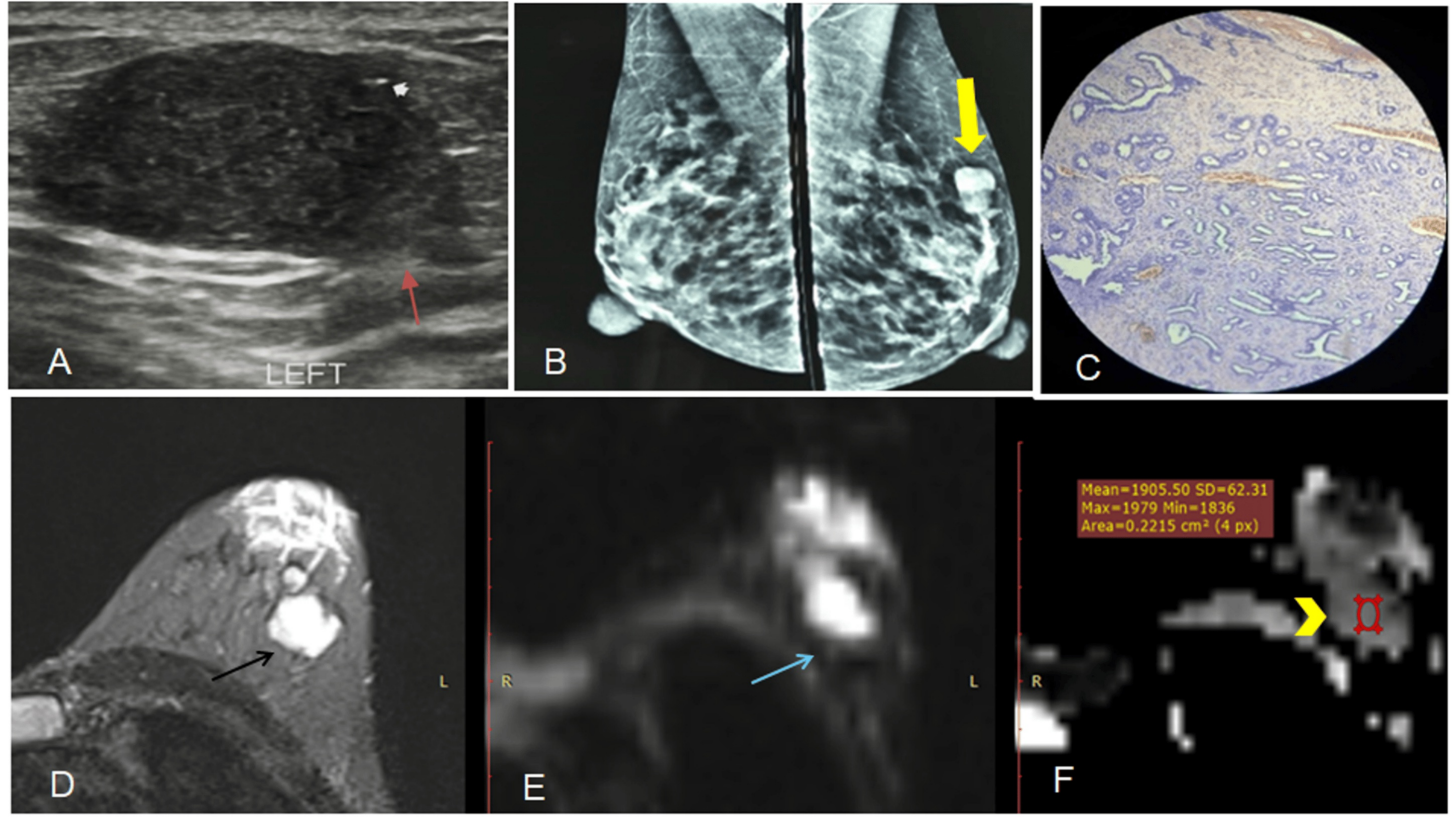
Fibroadenoma (A) Ultrasound shows an oval, hypoechoic lesion with intralesional calcification (white arrow) and indistinct margins (red arrow), categorized as BI-RADS IVa.
